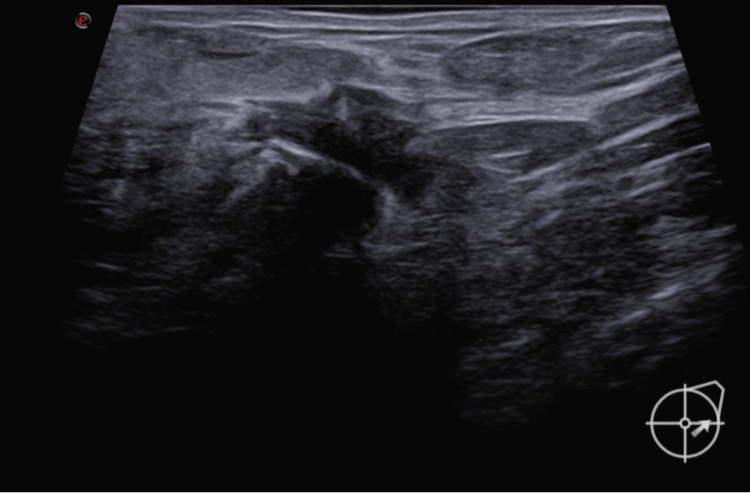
RFID usage: post-deployment Once the needle is in a good position, the RFID is deployed within the tumour under ultrasound guidance, as seen in the figure. RFID: radio-frequency identifier device
